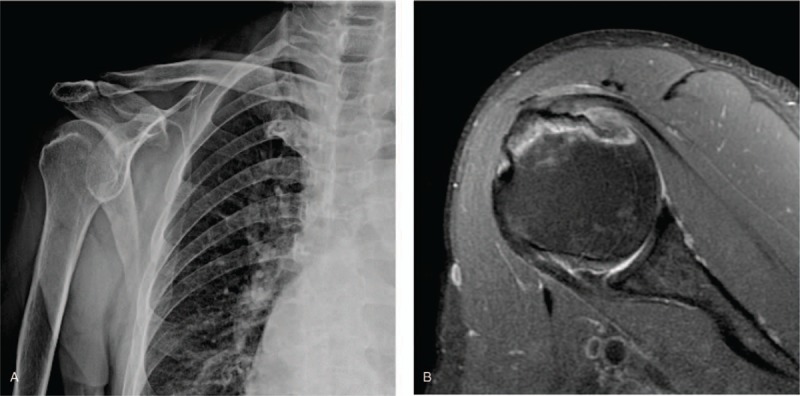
(A): A focal wedge-shaped sclerosis on the posterior aspect of the right greater tuberosity. (B): Right shoulder magnetic resonance imaging of the biceps axial view. Proton density fat-suppressed image shows a double-line sign involving the greater tuberosity, posterior and superior aspect of humeral head, a lesion compatible with osteonecrosis.
